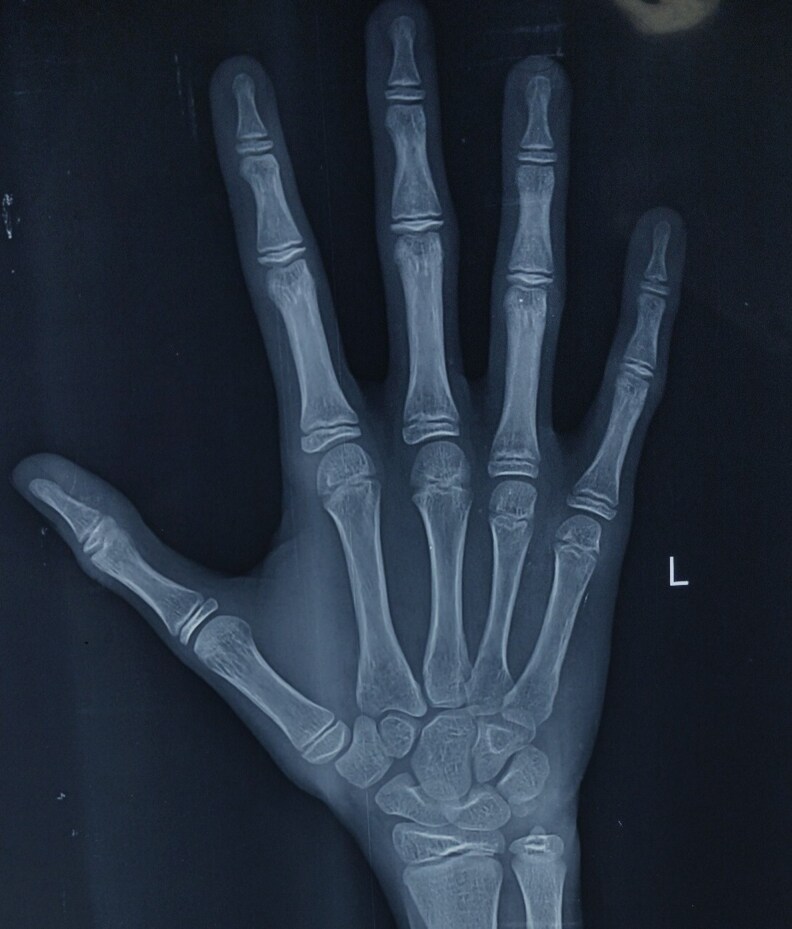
X-ray of left hand and wrist is suggestive of a bone age of approximately 11 to 12 years according to the Greulich and Pyle bone age estimation atlas.
